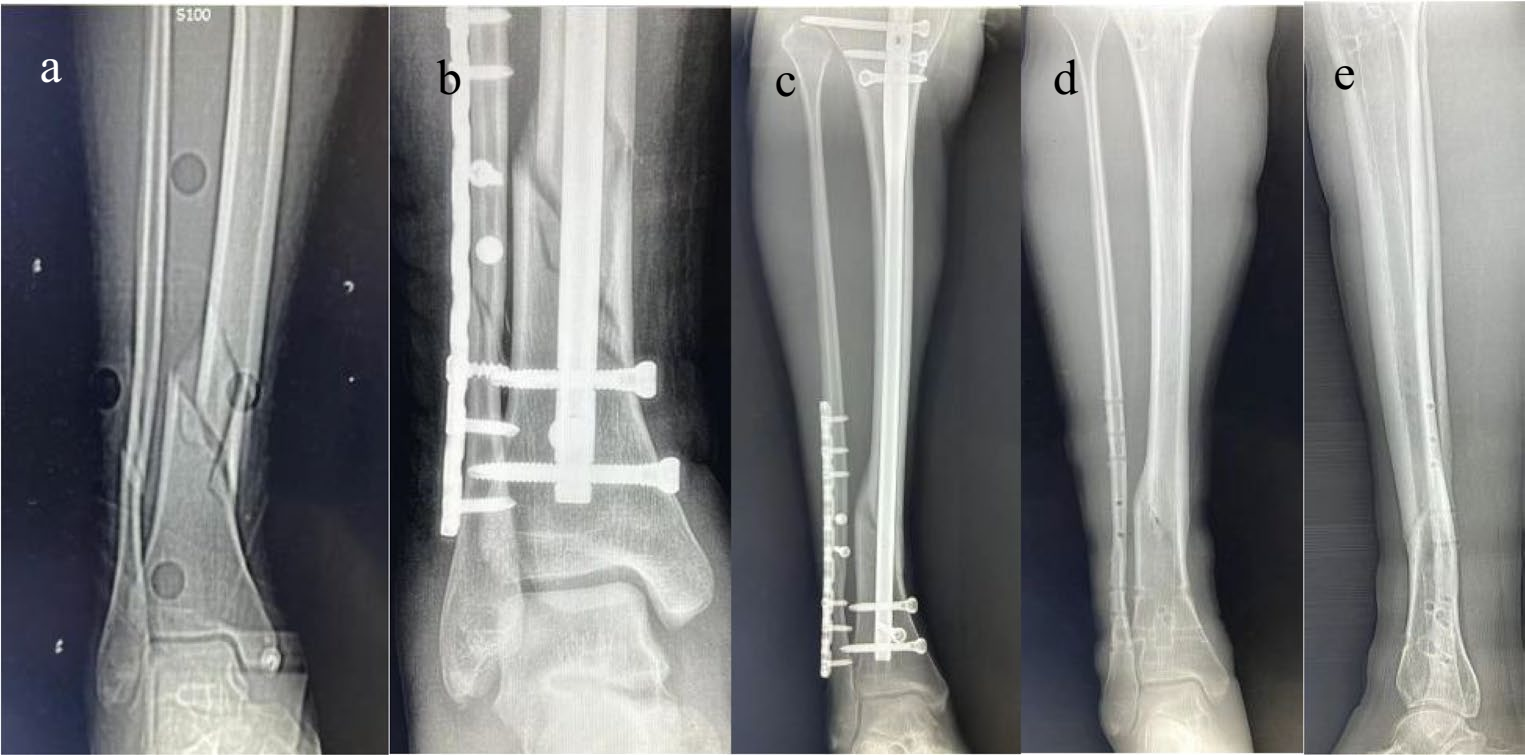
Distal tibial fracture, poor alignment after intramedullary nailing, 1-year follow-up, malunion radiographs. (a) Preoperative radiographs with a TFI ratio of 1.99; (b) postoperative reexamination of the radiographs showed poor alignment, and the alignment of the anteroposterior radiographs turned inward at an angle of 7°; (c) 5 months after surgery, the fracture line appeared slightly blurred; (d, e) fracture malunion occurred after the internal fixation device was removed
